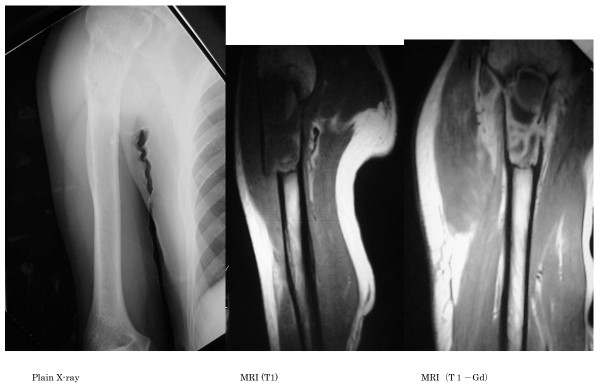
Plain X-ray radiographs and MRI on the first examination. Osteolytic hypertranslucency accompanied by pathological fracture was observed in the proximal humerus on plain radiographs. The periosteal reaction was not severe. On MRI, low intensity was detected on T1-weighted imaging, while cyst-like changes and low intensity of the tumor content were seen on T1 gadolinium contrast imaging.
